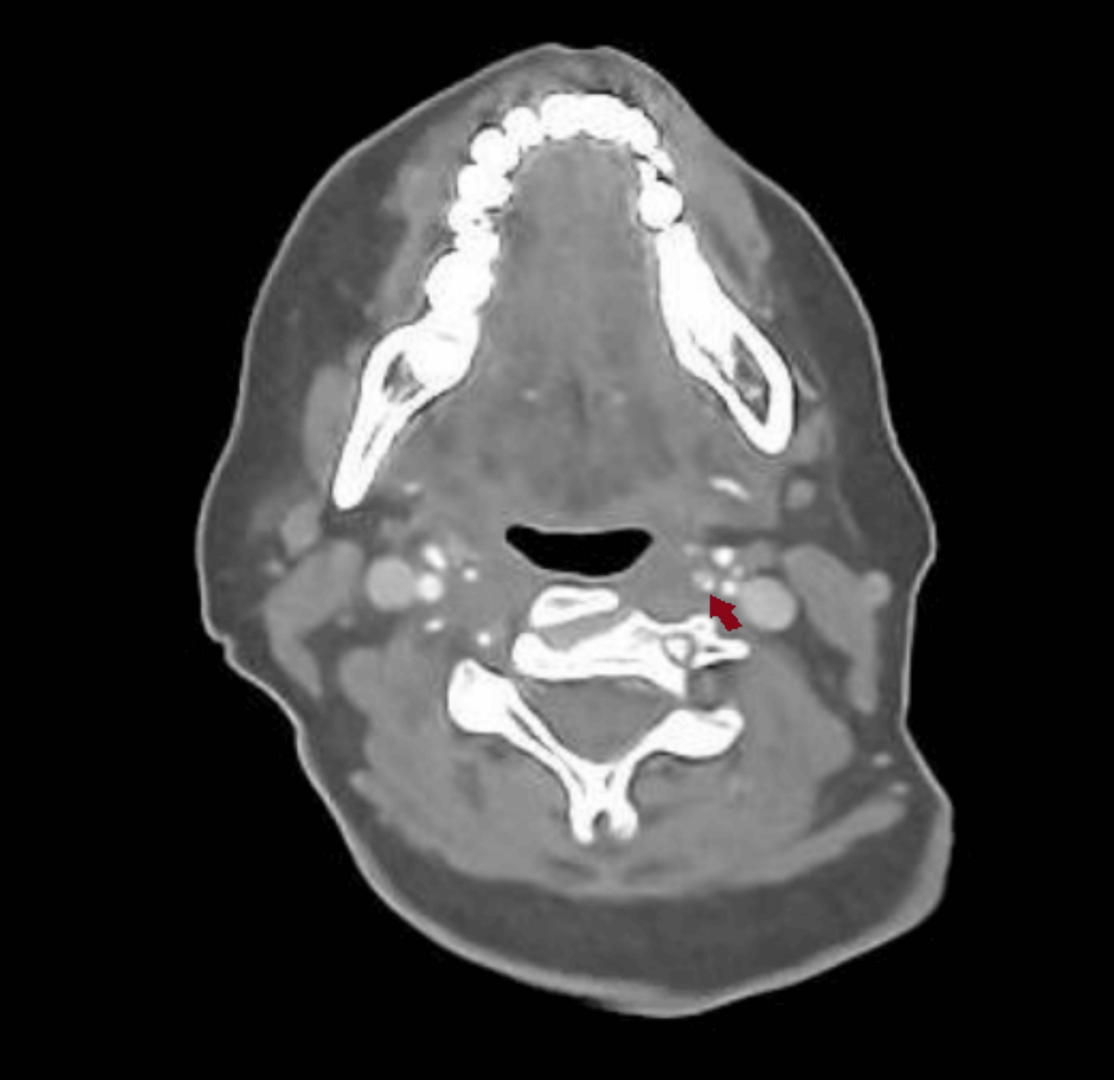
Axial CT carotid angiogram showing a narrow left internal carotid artery segment at the origin.
